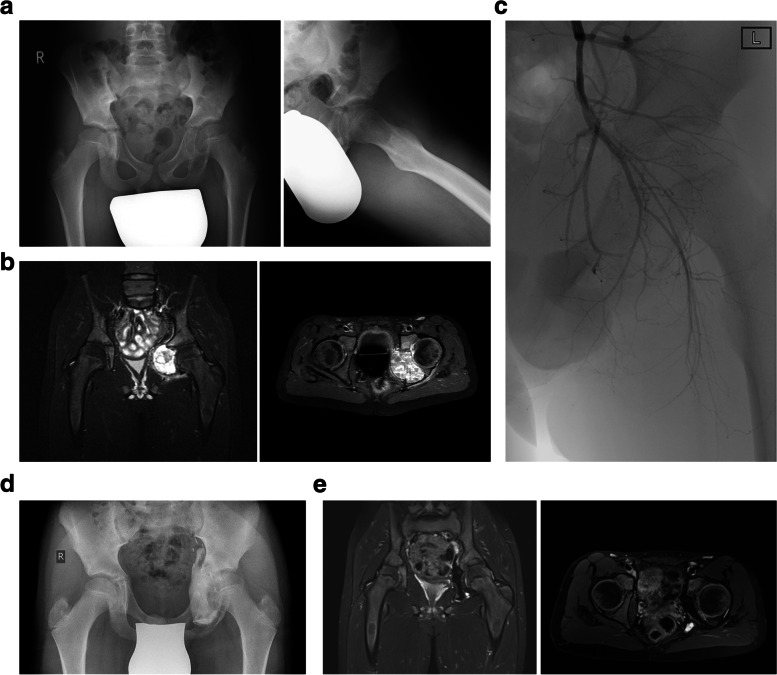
a-e Manifestation of an ABC in the left ilium and ischium: simple radiograph (a) and MRI scan (b); preoperative angiography without embolization (c); radiograph after intralesional curettage and cement augmentation (d), MRI scan 24 months after operation with small residual cystic element (e)
